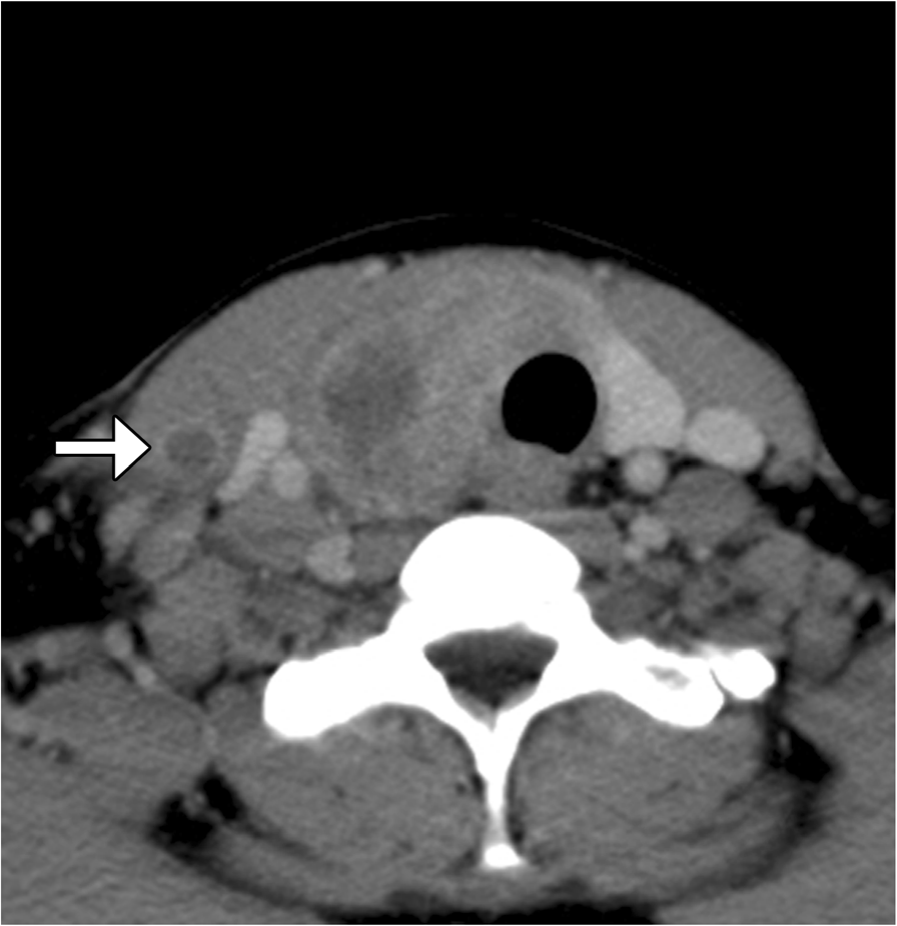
NUT carcinoma. Axial CT image shows an infiltrative mass in the right visceral space with involvement of the thyroid gland and necrotic right neck lymphadenopathy (arrow)
